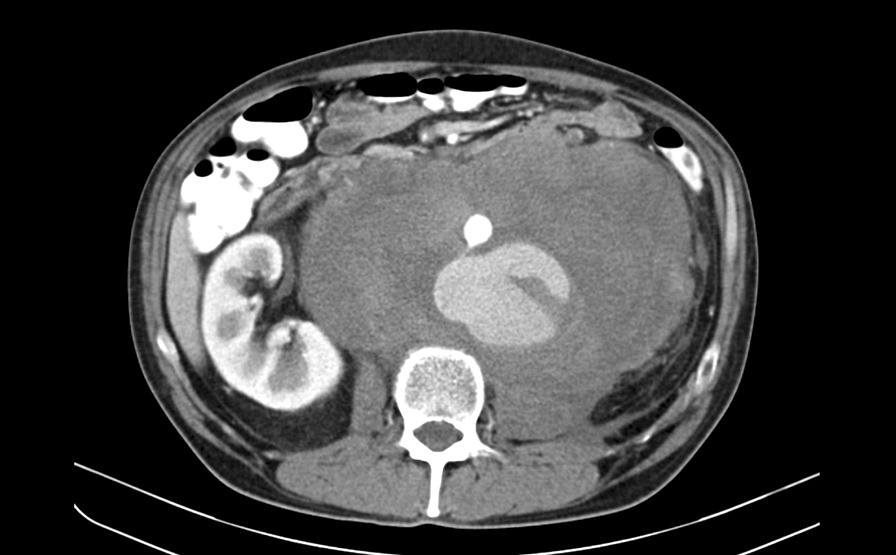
CT scan showed active extravasation of contrast agent into retroperitoneal tumor mass. ( transversal view)
